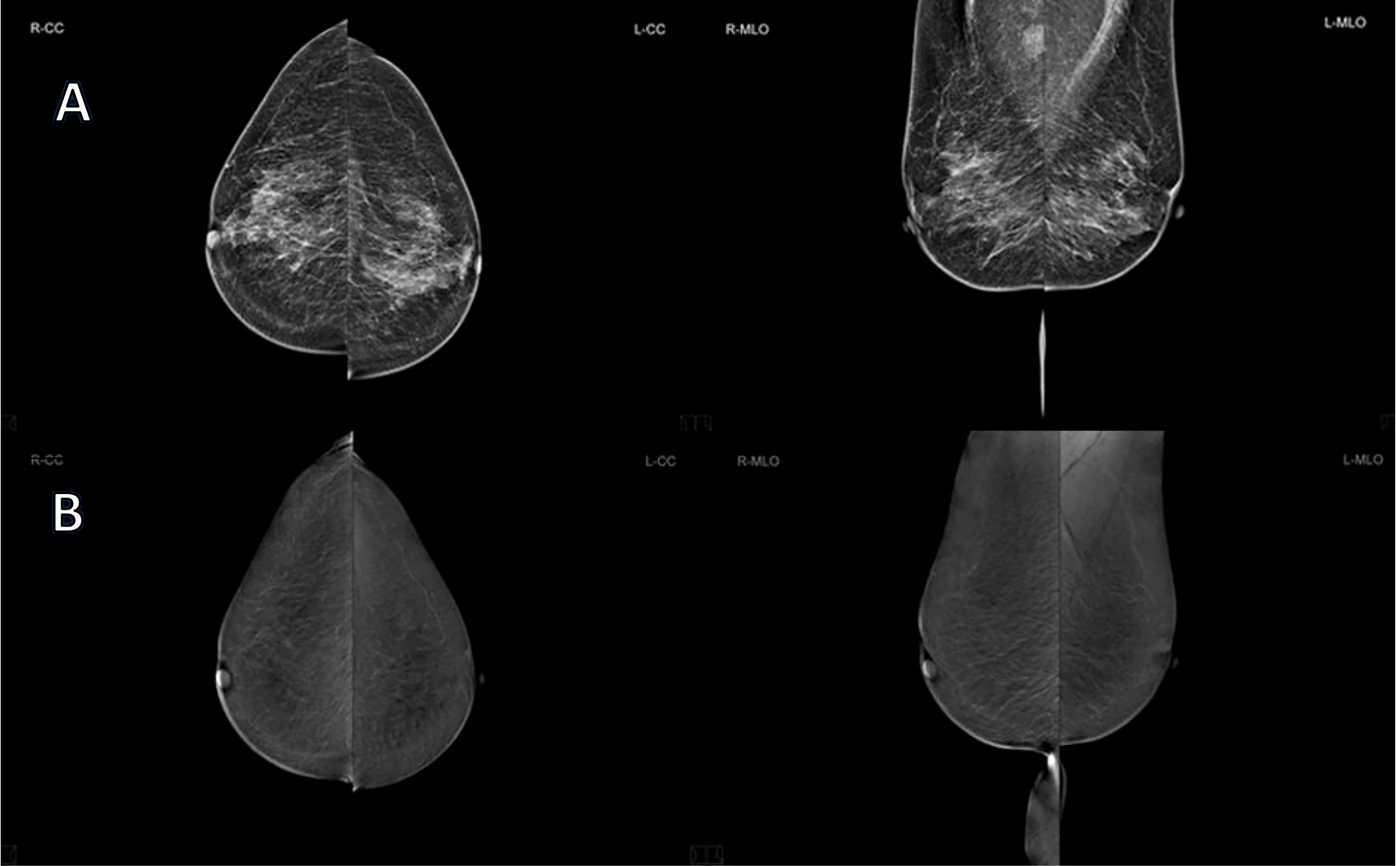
65 years old patient, with ACR type B breast density, recalled from screening due to the suspicious mass visible on RMLO in FFDM, classified as BIRADS 0 (A) and CEM (B) revealed no pathological enhancement. However, US was performed and it detected axillary lymph nodes with a typical metastatic appearance. One of them was biopsied and the pathology report revealed lymph node metastases of invasive lobular carcinoma. Breast MRI and PET-CT examinations were performed as well and they showed no signs of the primary tumor. The patient underwent neoadjuvant hormonal therapy and right mastectomy with axillary lymph node dissection. The final pathology report revealed multicentric invasive lobular carcinoma (grade 2) of the right breast with metastases to 23 out of 28 axillary lymph nodes.
